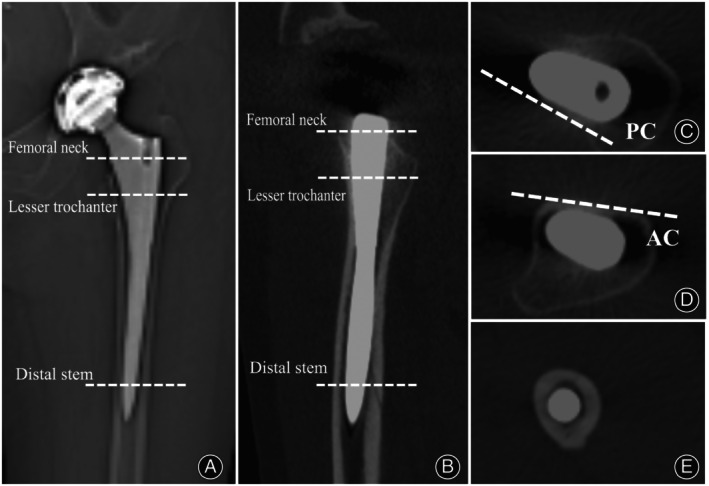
The fixation of the femoral stem in the sagittal plane: (A) The anterior to posterior image after total hip arthroplasty (THA); (B) Computed tomography (CT) reconstruction image in the sagittal plane after THA; (C) Posterior cortex (PC) at the femoral neck; (D) Anterior cortex (AC) at the lesser trochanter (LT); (E) The stem is circular or elliptical at the distal stem.
